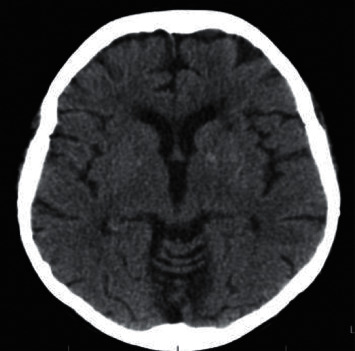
Cranial computed tomography showing previous left lacunar stroke.
